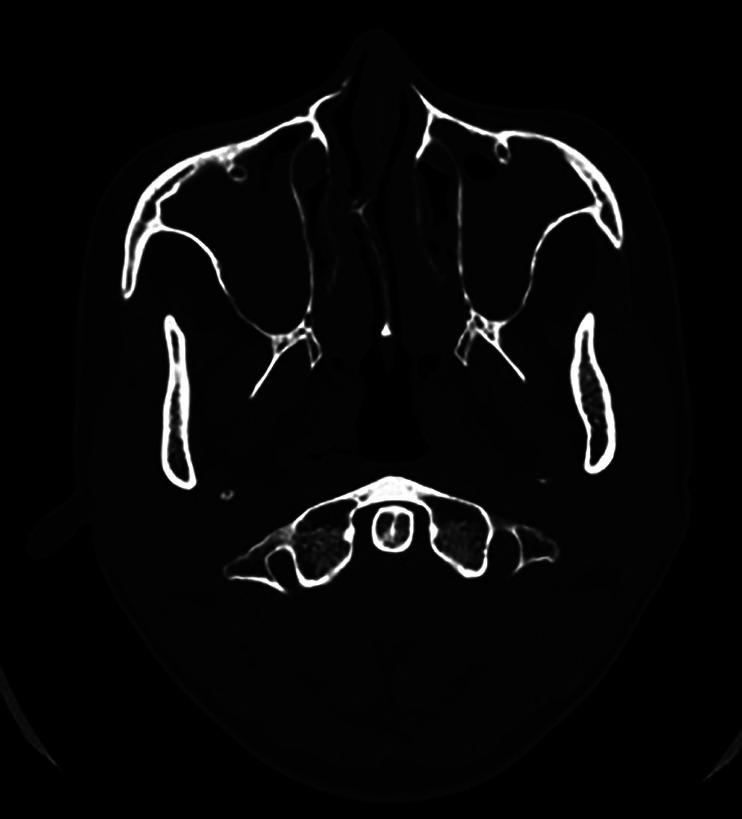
CT scan after 15 days showing the persistence of bilateral sinusopathy in an axial view.
